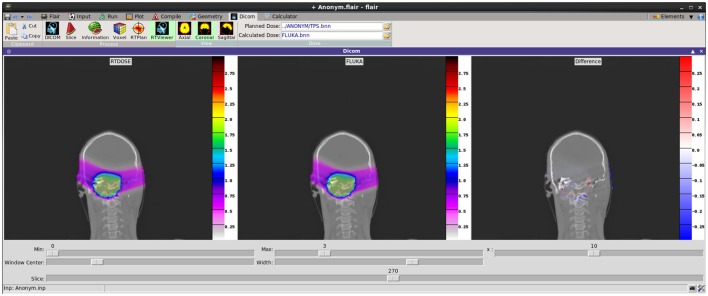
New DICOM Viewer interface. Coronal CT plane with mapped physical dose [Gy] from RTDOSE and FLUKA calculation. On the right differences between obtained values [Gy].
